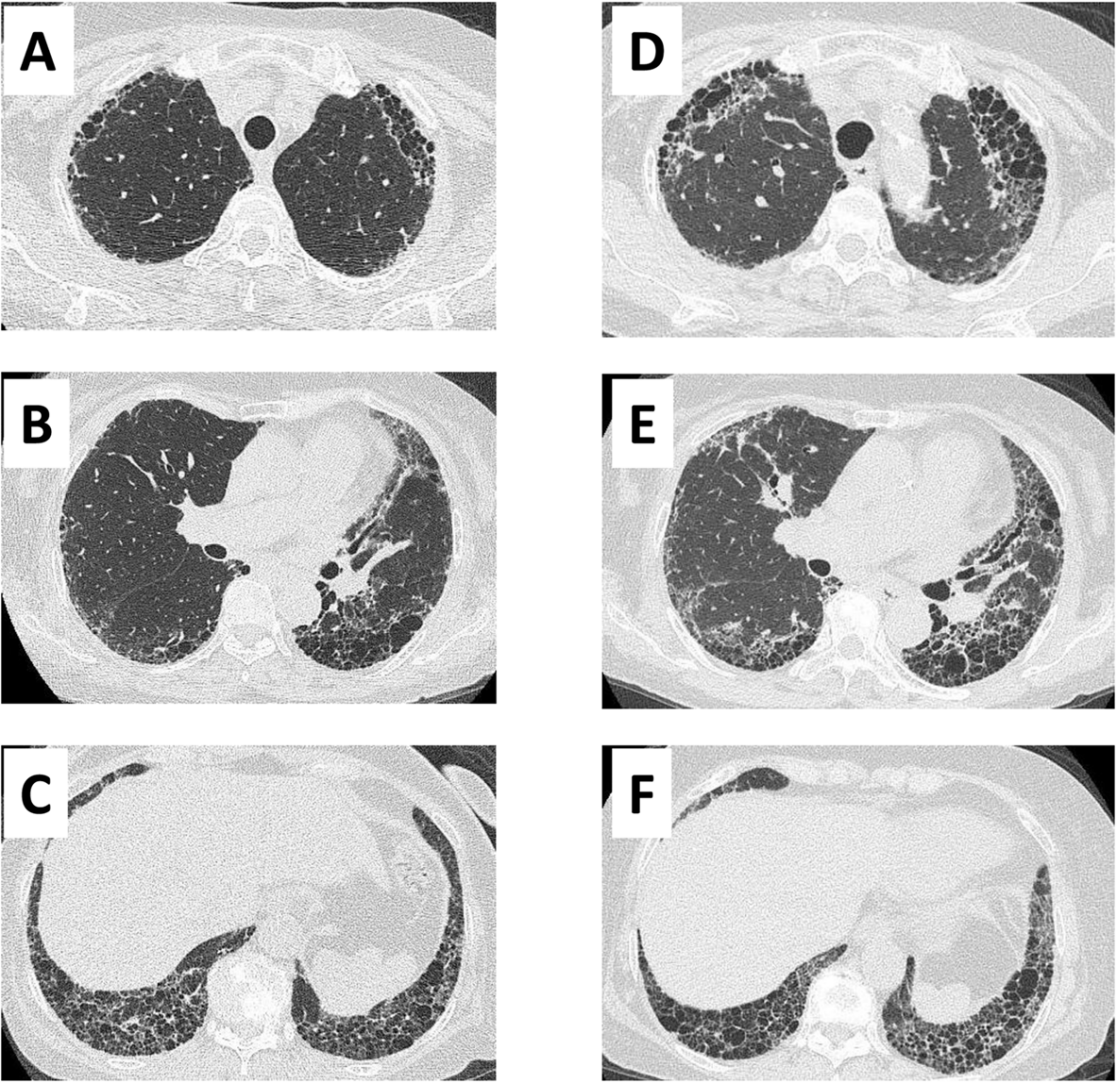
Representative thin-slice CT images of the lung from a patient with ANCA nephritis with a UIP pattern. (a–c) CT images at initial examination. (d–f) CT images 48 months after the initial CT examination. CT, computed tomography; ANCA, anti-neutrophil cytoplasmic antibody; UIP, usual interstitial pneumonia
